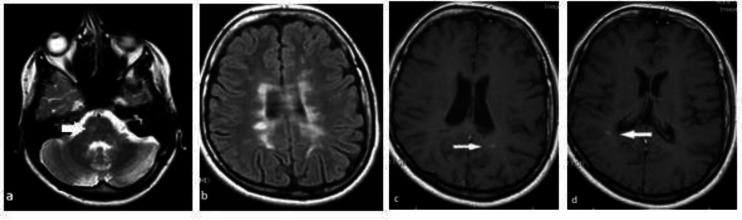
Axial views of the T2 weighted and FLAIR (a and b) and T1 weighted imaging with contrast (c and d) of the brain MRI. A new T2 lesion in the right side of the pons (thick white arrow) and 2 gadolinium enhancing lesions (thin white arrows) in the both sides of the centrum semiovale are seen
